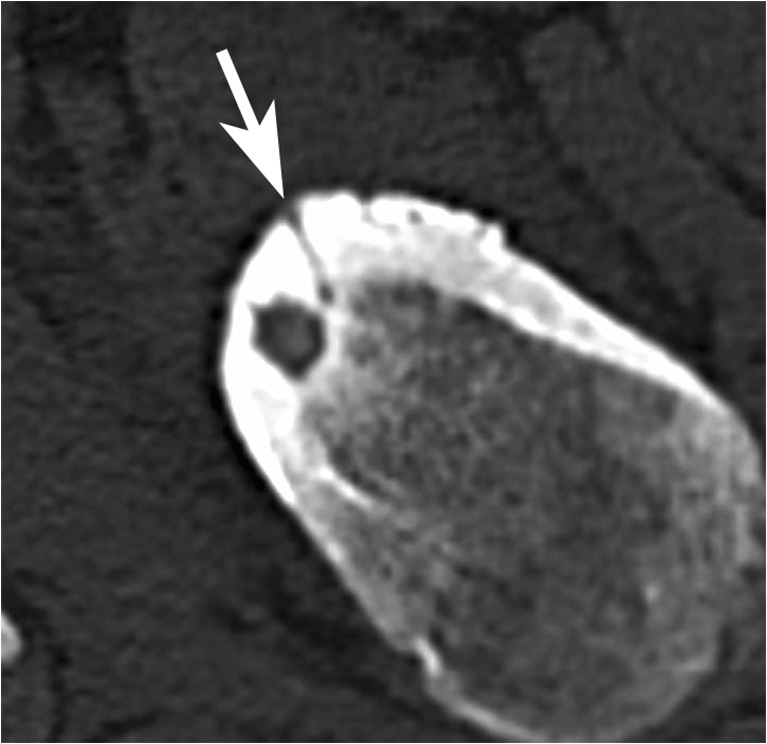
Axial CT image of a femoral neck OO demonstrates hypertrophic vascular channel (arrow) between the periosteal surface and the nidus area
